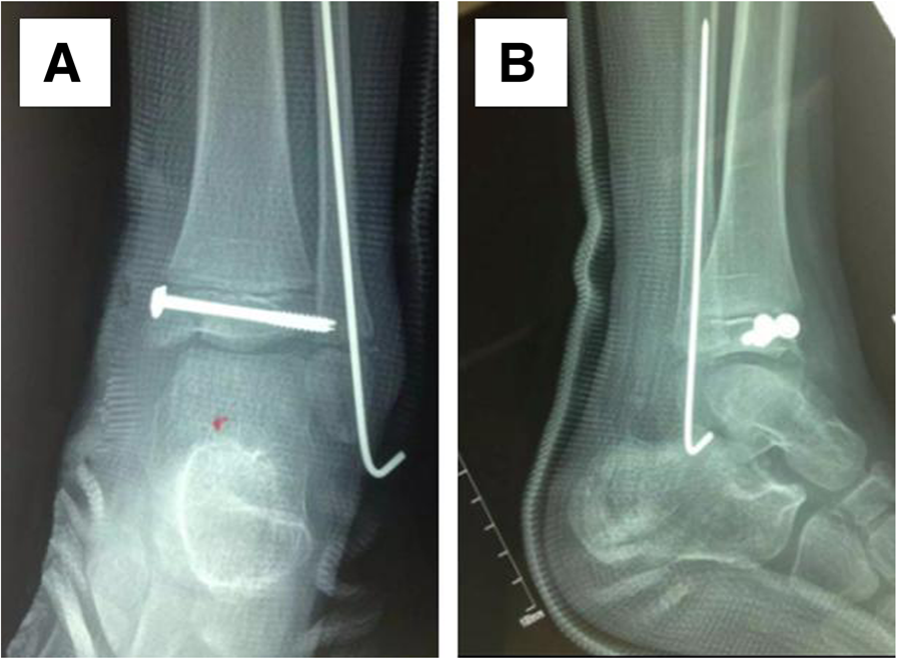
Early postoperative X-rays (a, the anteroposterior film, b, the lateral film) of the child in Fig. 1. The medial malleolus fracture was fixed with two cannulated screws, and the lateral malleolus fracture was fixed with a Kirschner wire along the radial axis
